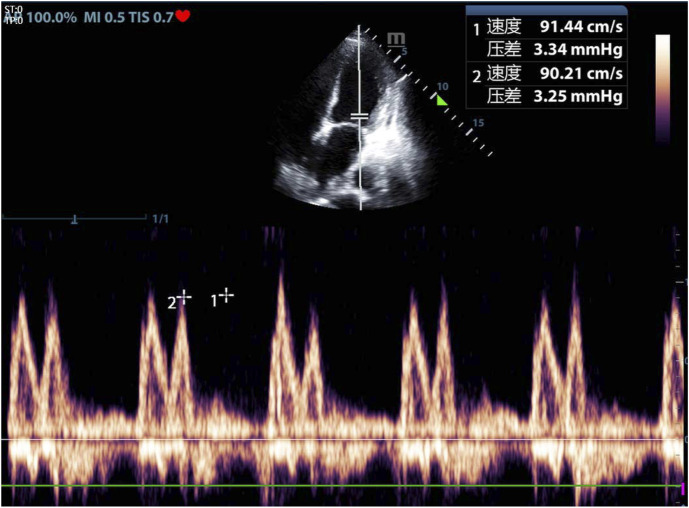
Echocardiography during SBT-T after reintubation. E/A: 1.0, E/e’: 16.2 (with permission from the patient.)
